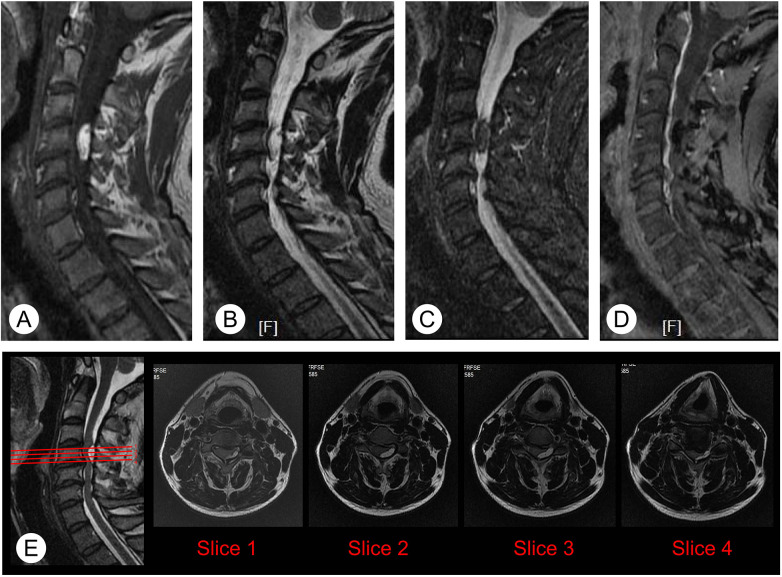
Magnetic resonance images of the cervical spine before surgery (A,B) T1- and T2-weighted images revealed a hyperintense lesion located posterior to the C4 vertebral body. (C) Fat-suppressed images showed the lesion appears hypointense with several internal linear signal voids. (D) Contrast-enhanced T1-weighted images similarly demonstrate hypointense of the lesion. (E) Axial T2-weighted images (slices 1−4) and their location image suggested the lipoma located entirely posterior to the C4 vertebral body.
